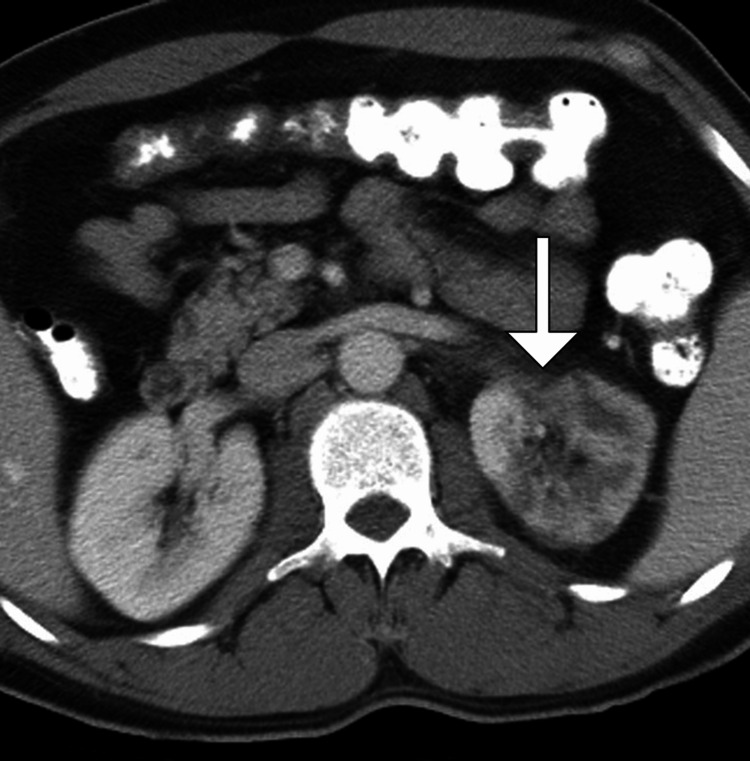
Axial contrast-enhanced CT image demonstrating a wedge-shaped area of decreased enhancement in the left kidney (arrow), consistent with an acute renal infarction CT: computed tomography
